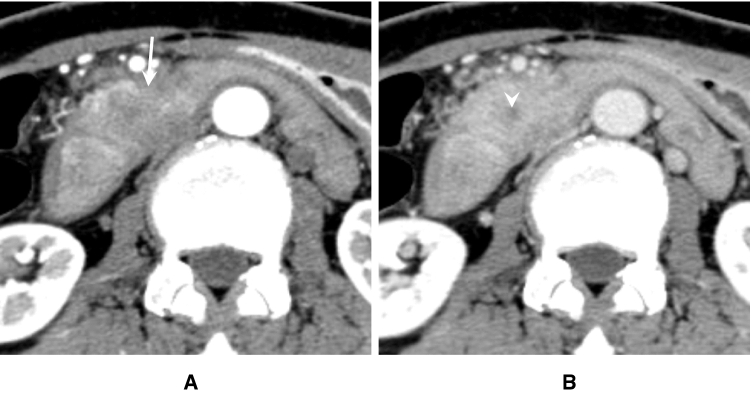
Contrast-enhanced CT images of pancreatic head cancer. a DCE-CT (pancreatic phase) shows a hypovascular lesion in the pancreatic head (arrow). b DCE-CT (delayed phase) reveals a pancreatic head lesion with inhomogeneous delayed enhancement (poor enhancement in the central area; arrowhead)
